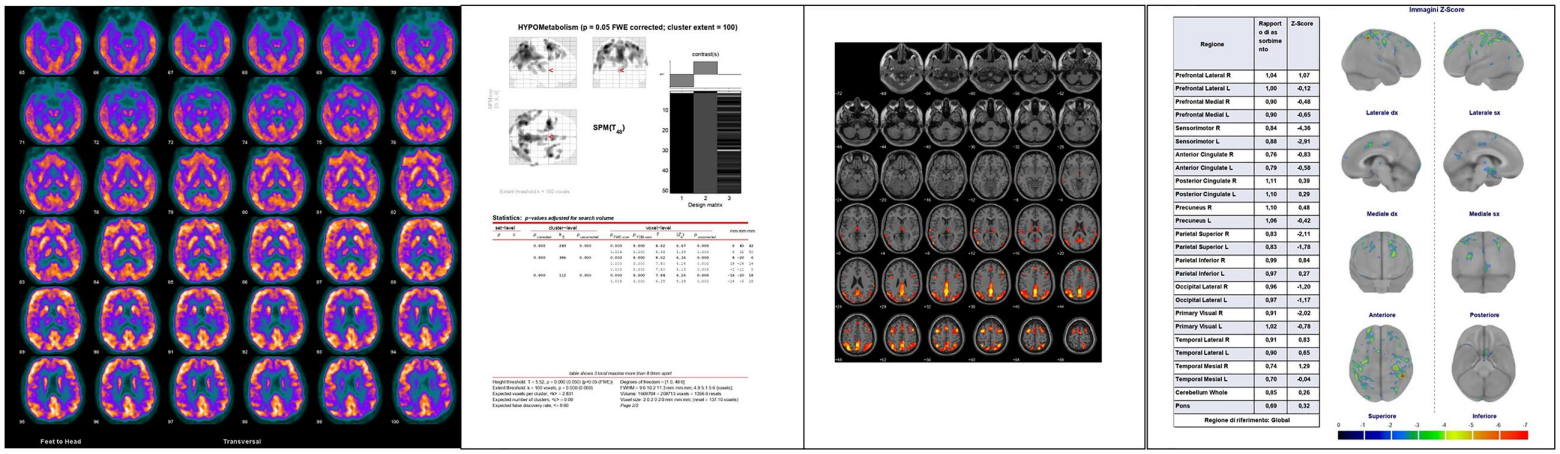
Examples of output from the three FDG-PET scan rating methods for an amyloid-positive patient with an amnestic presentation (from left to right): display for visual interpretation of uptake images; INLAB glass brain and statistical map; output of Cortex ID Suite
